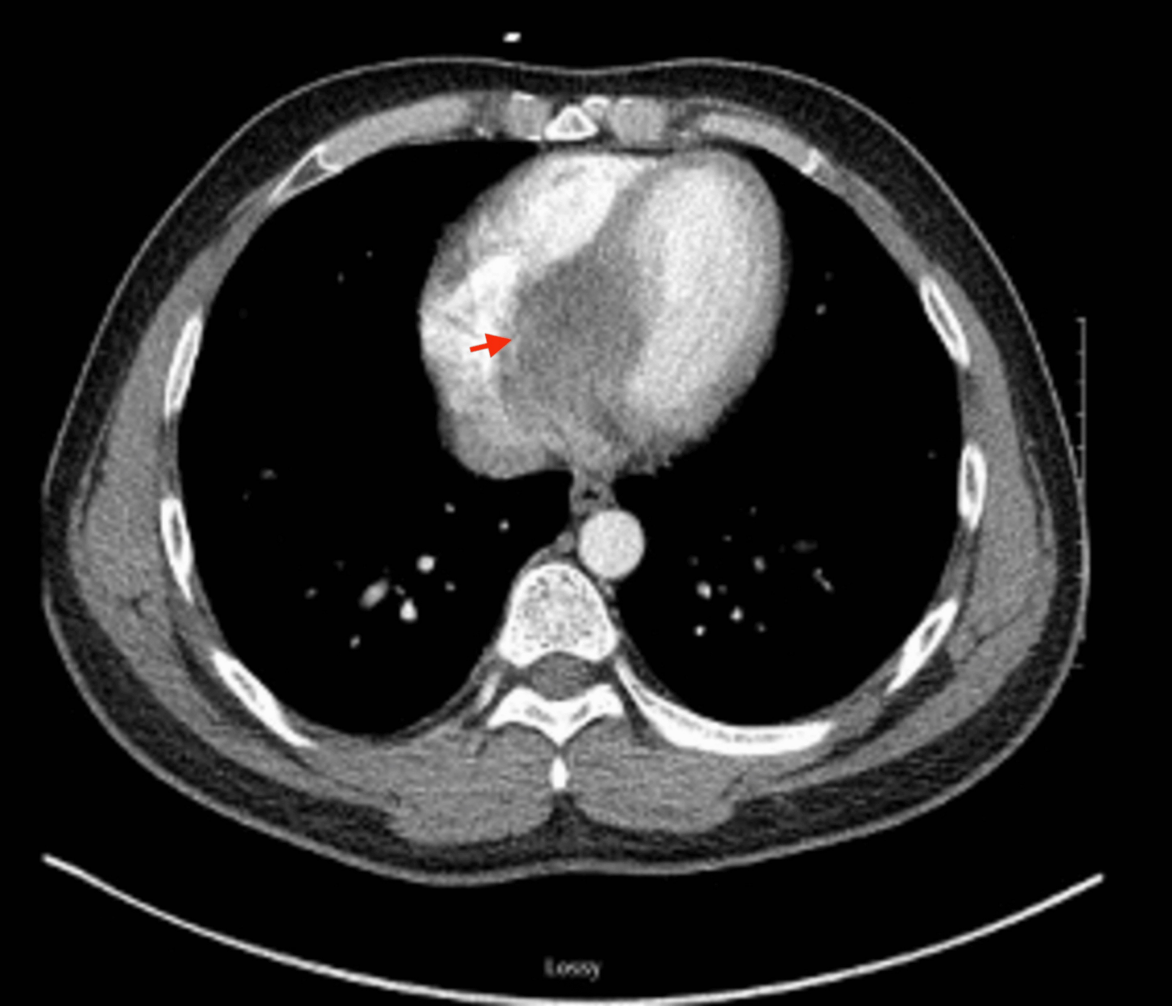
Non-gated chest CT showing a large hypoattenuating mass in the interventricular septum
